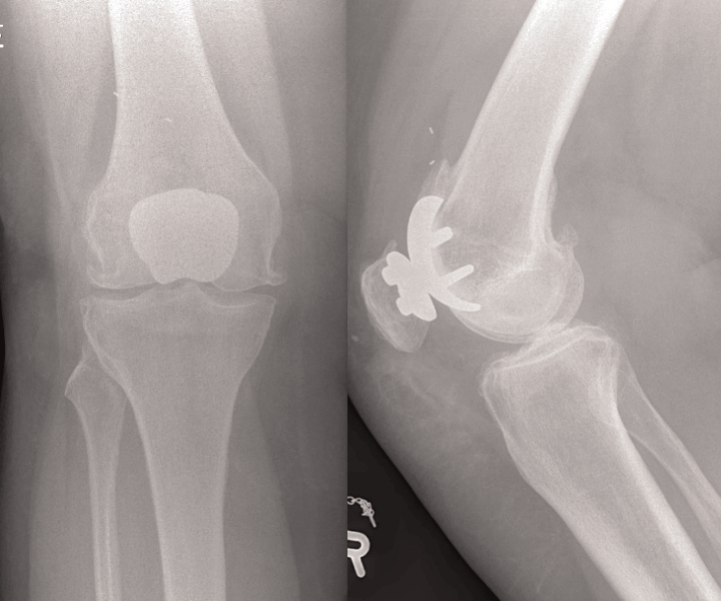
Radiograph showing dissociation of polyethylene patellar bearing. The femoral component of the LCS patellofemoral prosthesis is positioned in moderate flexion with increased thickness of the prosthesis-patella construct. The femorotibial compartment demonstrates osteophytes and narrowing of joint space.
